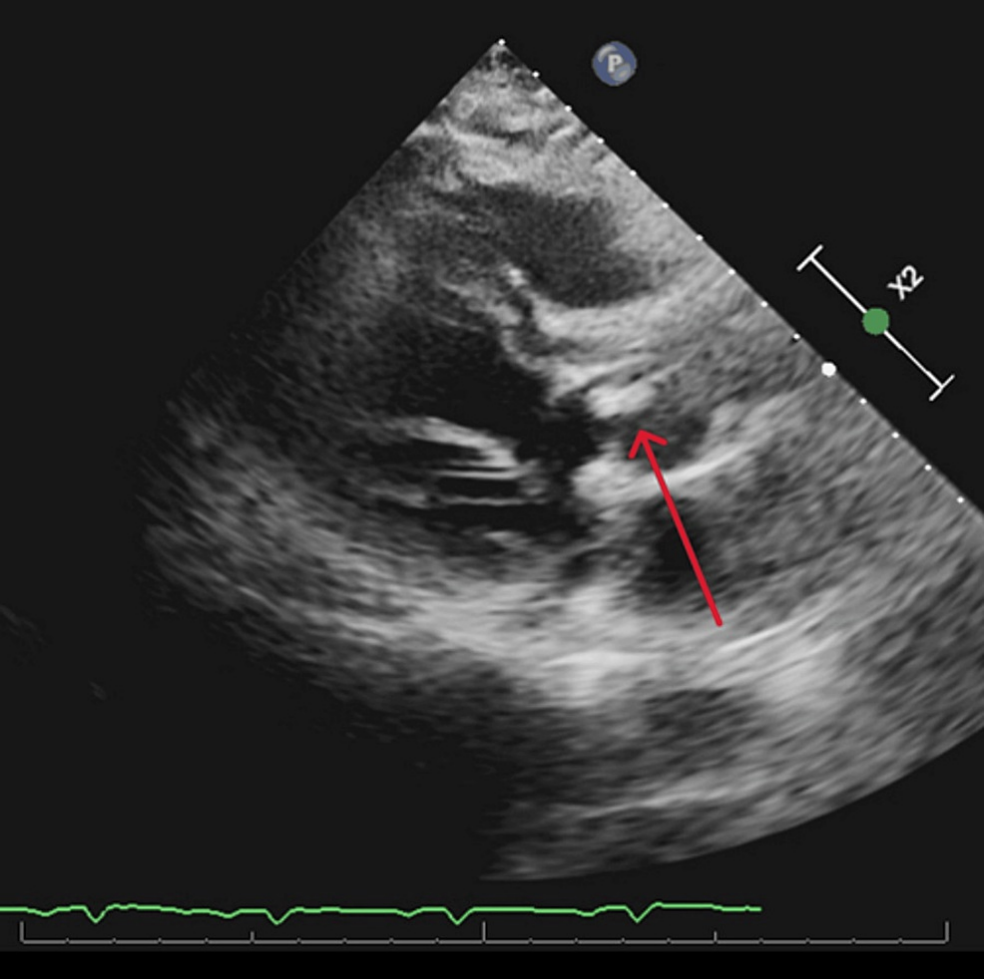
BAV vegetation on transthoracic echocardiogram BAV: bicuspid aortic valve
